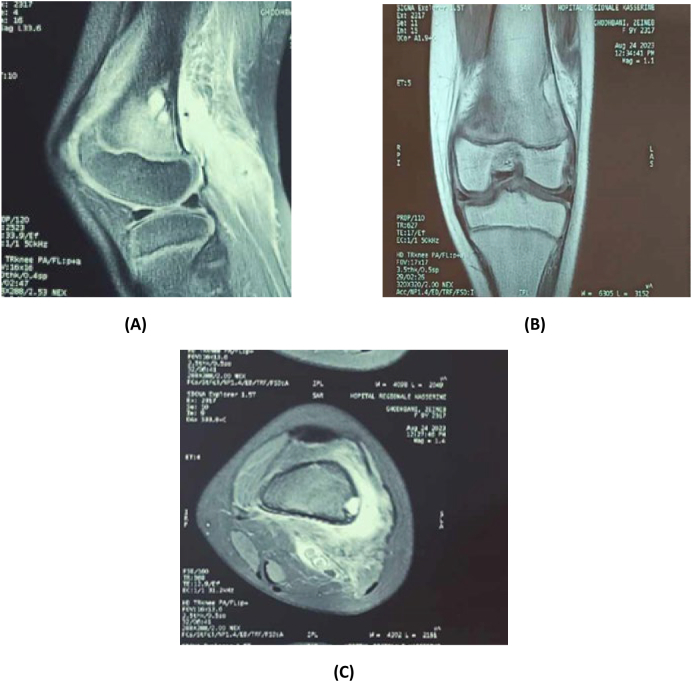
Knee MRI: A and C: T1 MRI sequency B: T2 MRI sequency MRI revealed a multilocular cystic lesion measuring 5 cm, arising from the lateral cortex of the distal femoral metaphysis, with no evidence of soft tissue extension.
